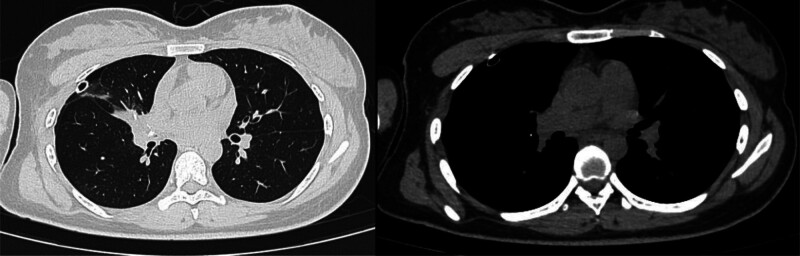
December 05, 2023 chest CT. CT = computed tomography.
